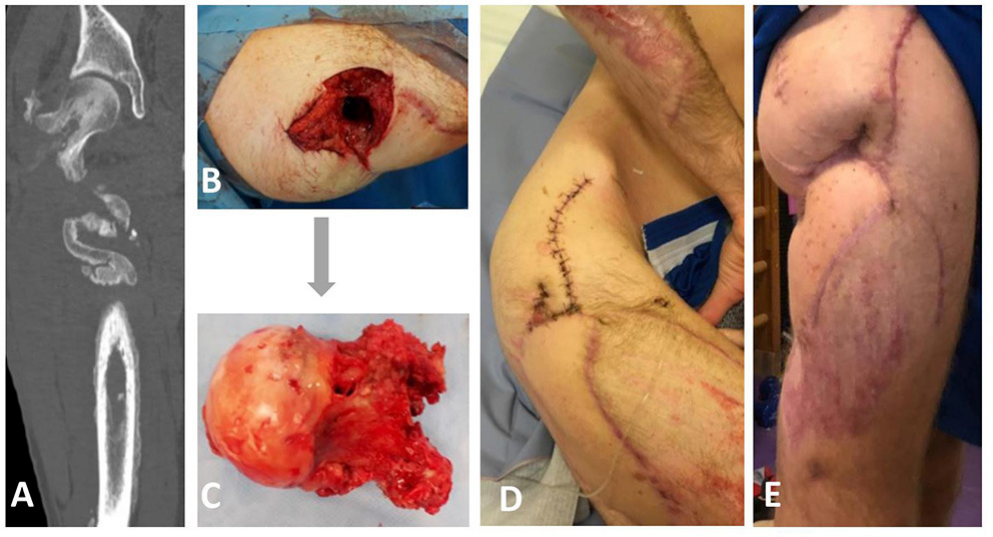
Clinical and radiological appearance of the patient prior, during, and after bacteriophage therapy. (A) Computed tomography revealing a fistula that connects femoral head and skin on the right upper third of the lateral femur surface. (B,C) Intraoperative findings from the right femoral head excision on the 5th of December revealing the presence of a femoral fistula. (D) Postoperative photograph showing irrigation system for local bacteriophage application. (E) The appearance of the wound two months after bacteriophage therapy.
